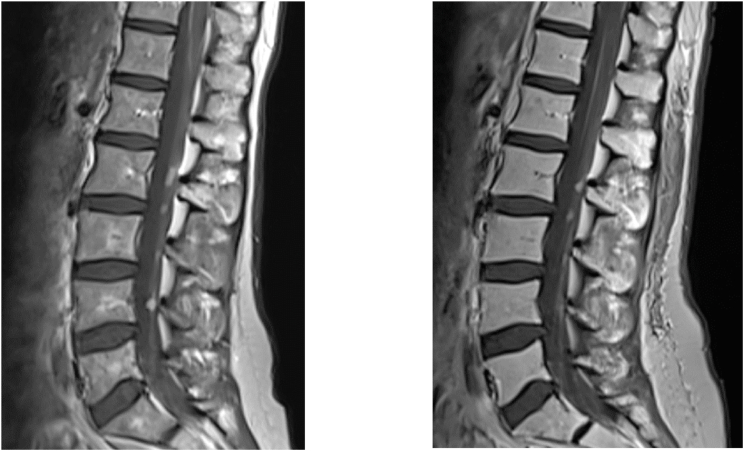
Extramedullary spinal metastases on sagittal T1 MRI with contrast. Before (left) and 6 months after radiotherapy (right).
